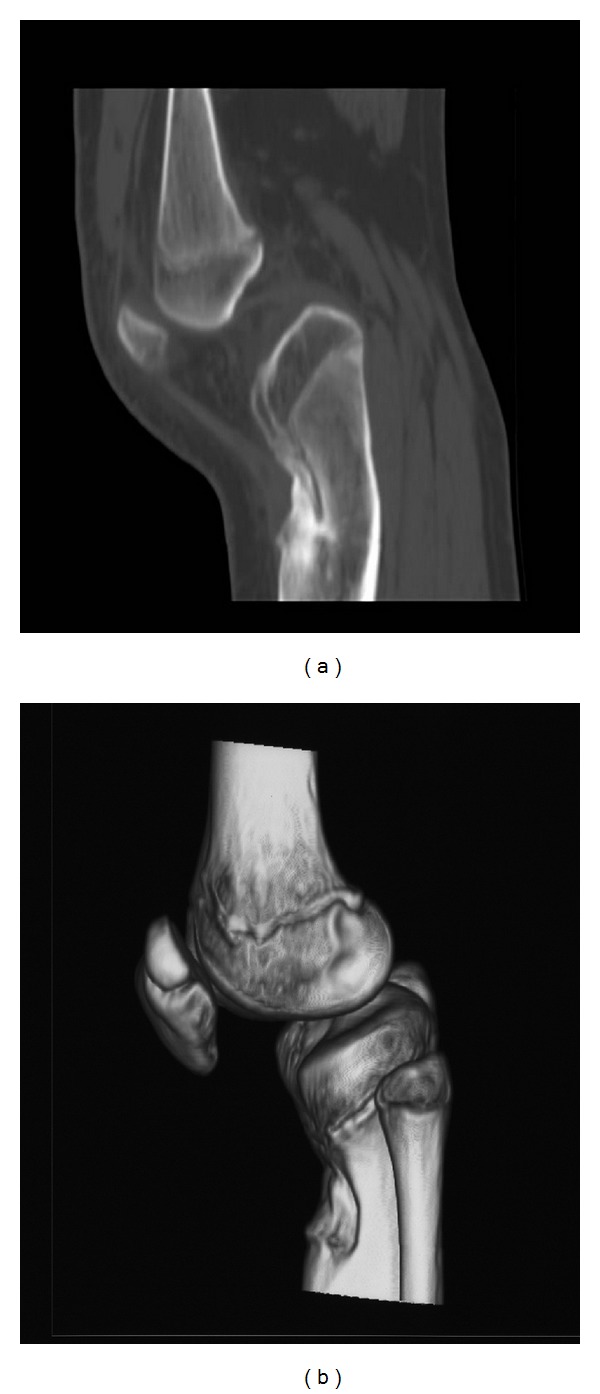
CT tomography of the left knee: posterior subluxation of the knee and significant deformity of the tibial tubercle.
